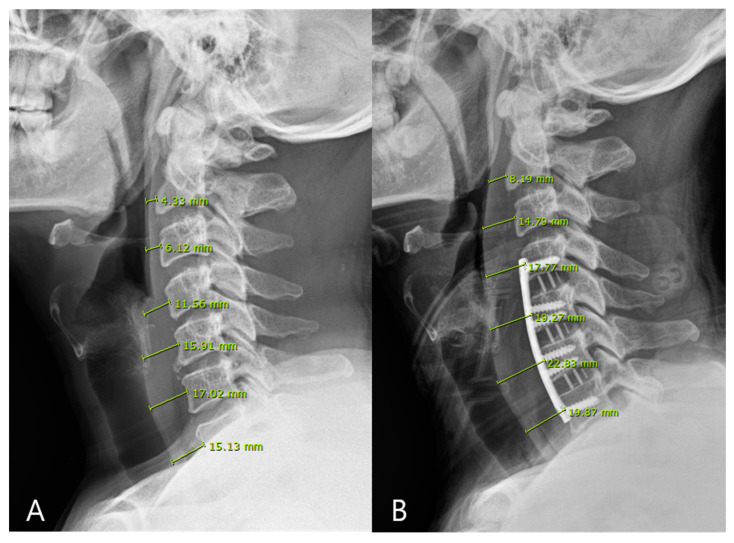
Prevertebral soft tissue swelling (PSTS) was calculated from the C-spine lateral radiographs. The sum of total variation in PSTS at each level from C2 to C7 was used to evaluate the relationship between PSTS and degree of dysphagia. (A) is preoperative PSTS at each level and (B) is postoperative PSTS at each level.
